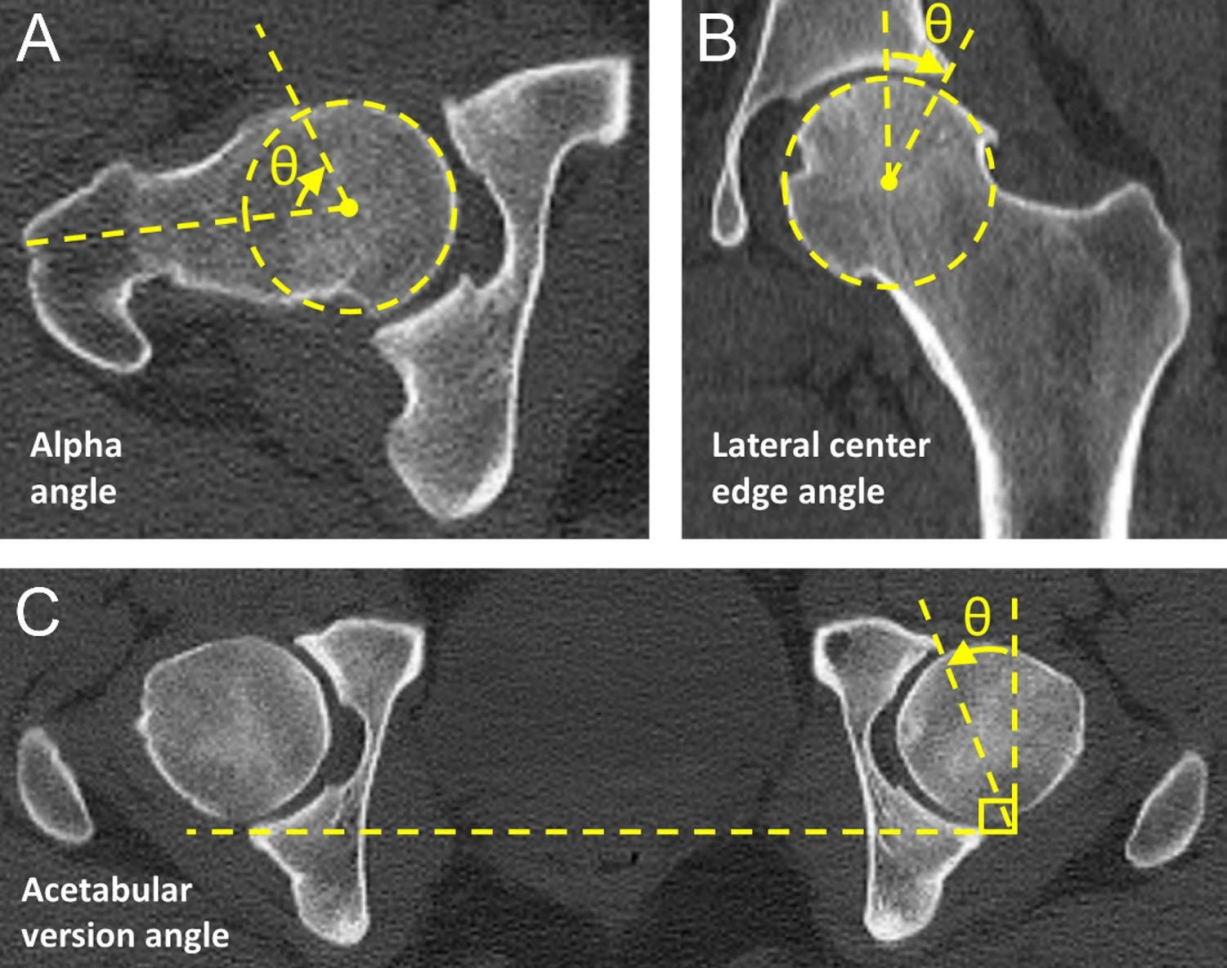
Assessment of morphometry on CT scans. (A) Alpha angle was measured between a line connecting the centers of the femoral head and its neck and a line from the center of the femoral head to where the distance from the center of the head exceeded its radius. (B) Lateral center edge angle was measured as the angle between the vertical axis of the pelvis and a line connecting the center of the femoral head and lateral acetabular margin. (C) Acetabular version was measured by a line drawn joining the posterior corners of the bilateral acetabulum and tangential lines drawn from the posterior corner to provide a true sagittal line for each acetabulum. The angle between the sagittal line and a line connecting the anterior and posterior corners of the acetabulum was recorded as acetabular version angle.
